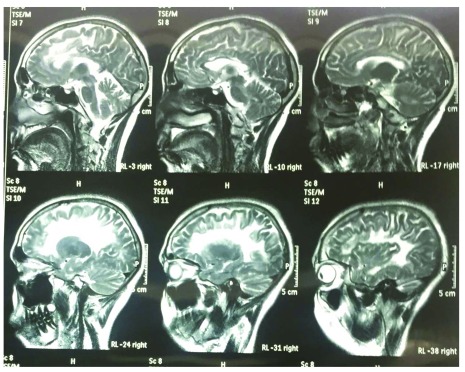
Saggital view of T-2 weighted images of the brain without FLAIR.
